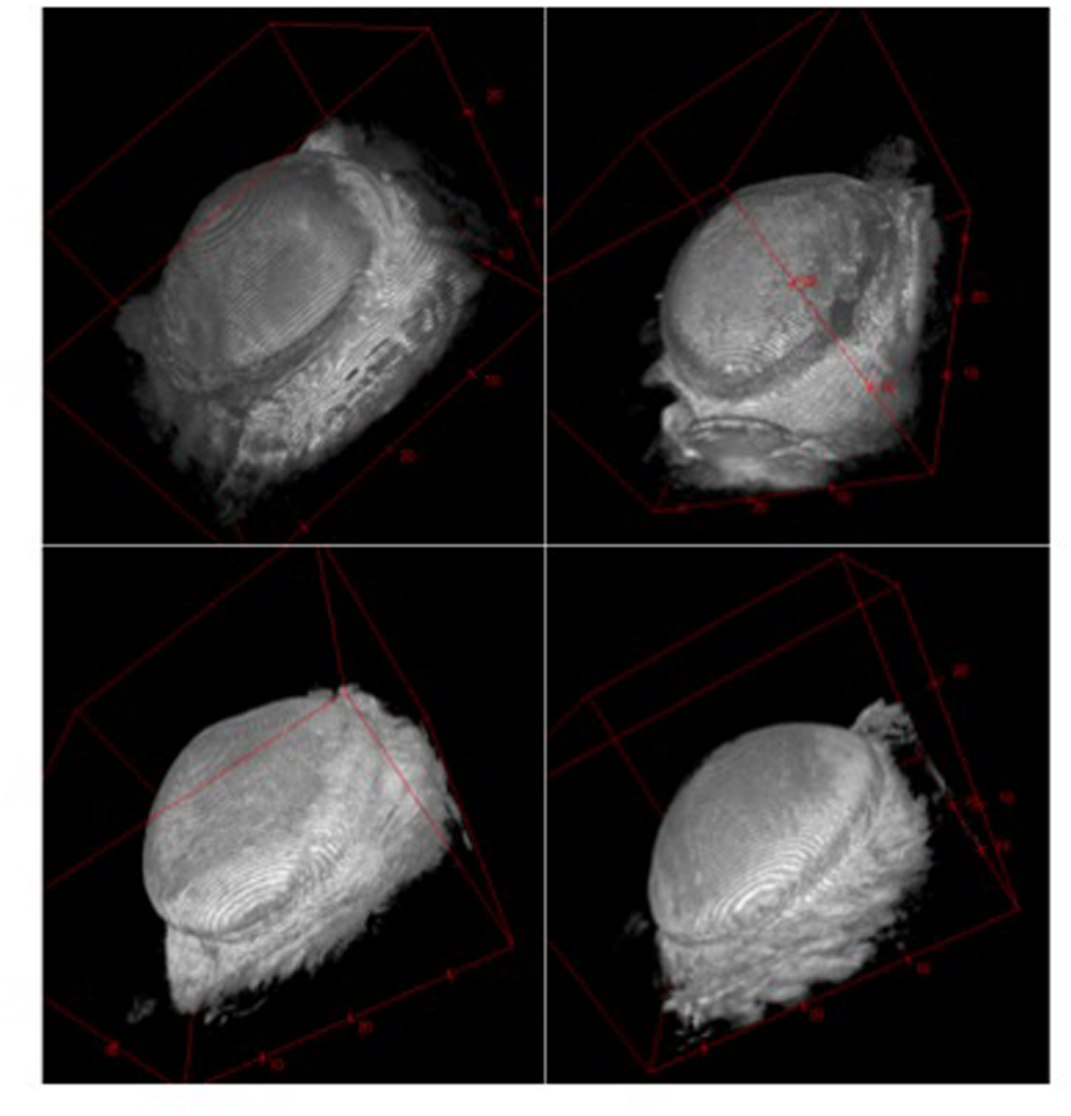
3D reconstruction of 7-Tesla MRI scans of smooth implants on day 60 (60s, upper left), textured implants on day 60 (60t, upper right), smooth implants on day 120 (120s, lower left) and textured implants on day 120 (120t, lower right).
